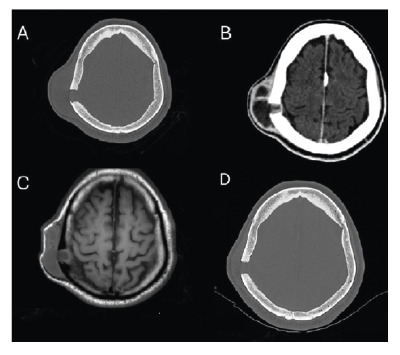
(A) Brain computed tomography, bone window, axial, showing a right parietal lytic bone lesion affecting the entire thickness of the shell: external board, diploe and internal board. There is also enlargement of adjacent soft tissues. (B) Brain computed tomography, parenchyma window, axial, showing fluid accumulation with peripheral enhancement in the soft tissues and involving the bone plane. (C) Brain magnetic resonance imaging (axial T1) showing fluid accumulation (hypointense on T1) in the soft tissues and in the bone of the right parietal region. (D) Brain computed tomography, bone window, axial, showing evident regression of adjacent soft tissues in the right parietal region after only three months of treatment.
